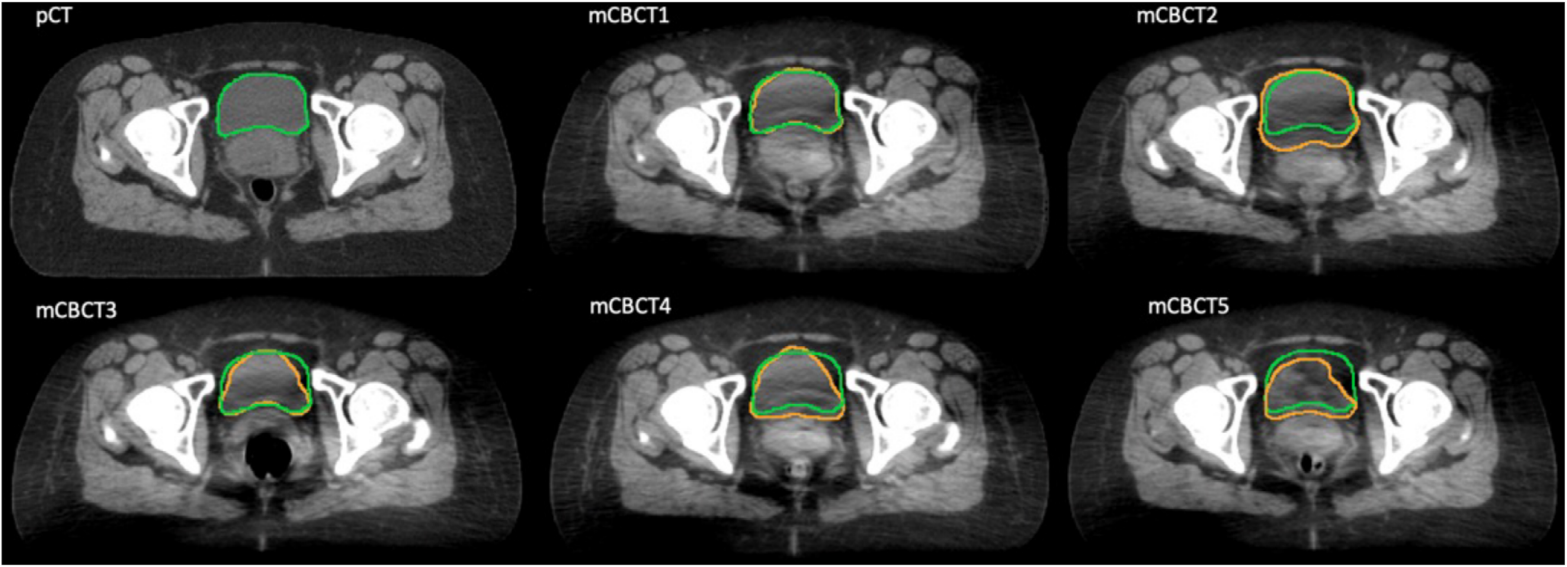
Patient 7 pCT and mCBCT1‐5 display the pCT bladder structure shown in green and each mCBCT‐specific bladder shown in orange. The variation in bladder filling and location between the weekly CBCT compared to the pCT is evident. The larger variation in bladder volumes degraded the DIR‐generated contours and required manual adjustments to be made.
